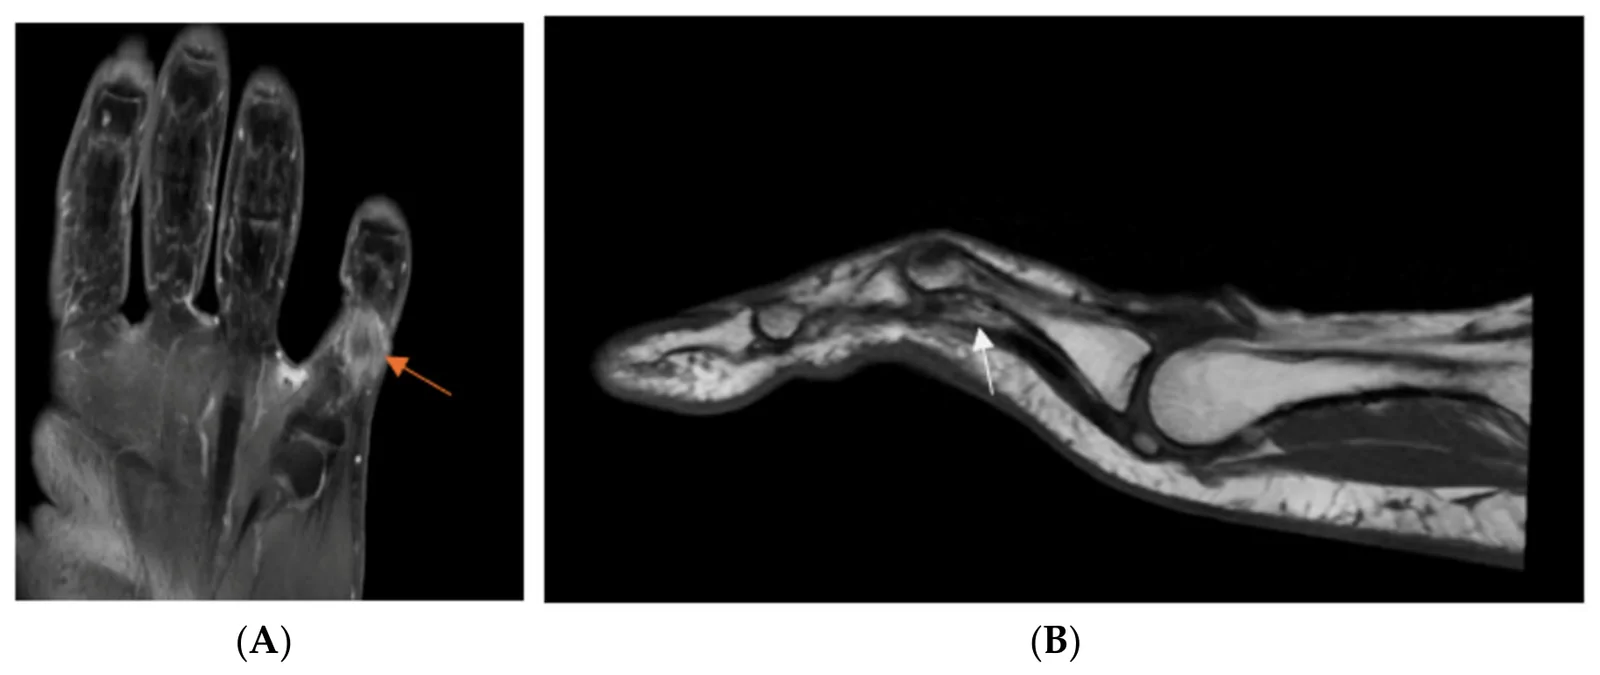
MRI ((A): T2—view and (B): T1—view) shows a solid nodular lesion (orange arrow) on the palmar surface of the proximal phalangeal of the little finger, which comes into direct contact with the flexor tendon sheath, mimicking Giant-Cell Tumor of the tendon sheath (white arrow).
